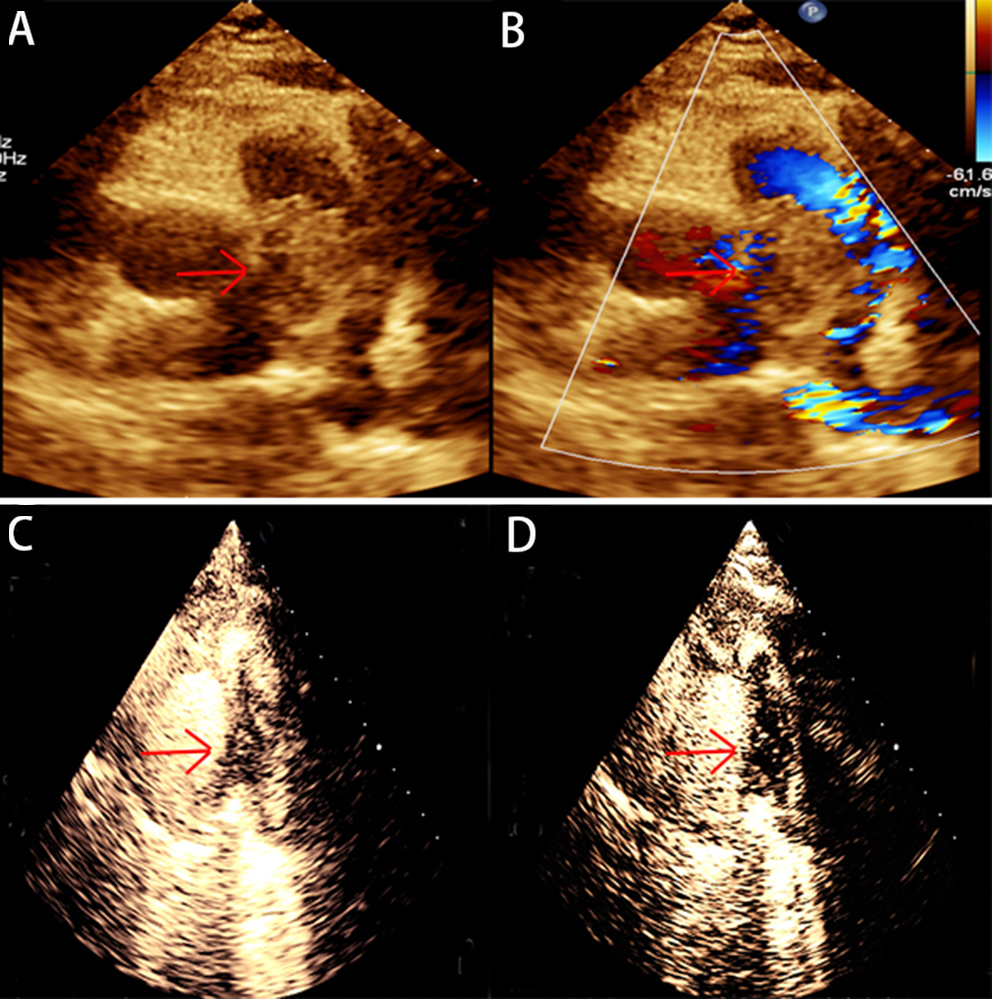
A, B Preoperative echocardiography: a large irregular hypoechoic mass detected within the main pulmonary artery. No blood flow revealed in the mass. C, D Contrast-enhanced echocardiography: the contrast agent was unevenly filling within the mass
